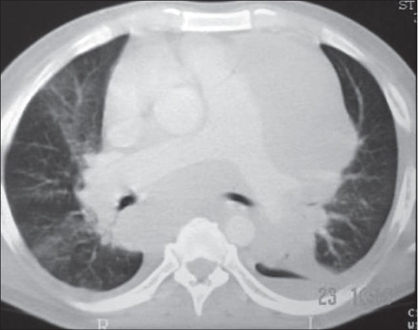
Patient 2: Chest computed tomography (CT) showing large mediastinal mass involving aortic arch and compressing main bronchi
